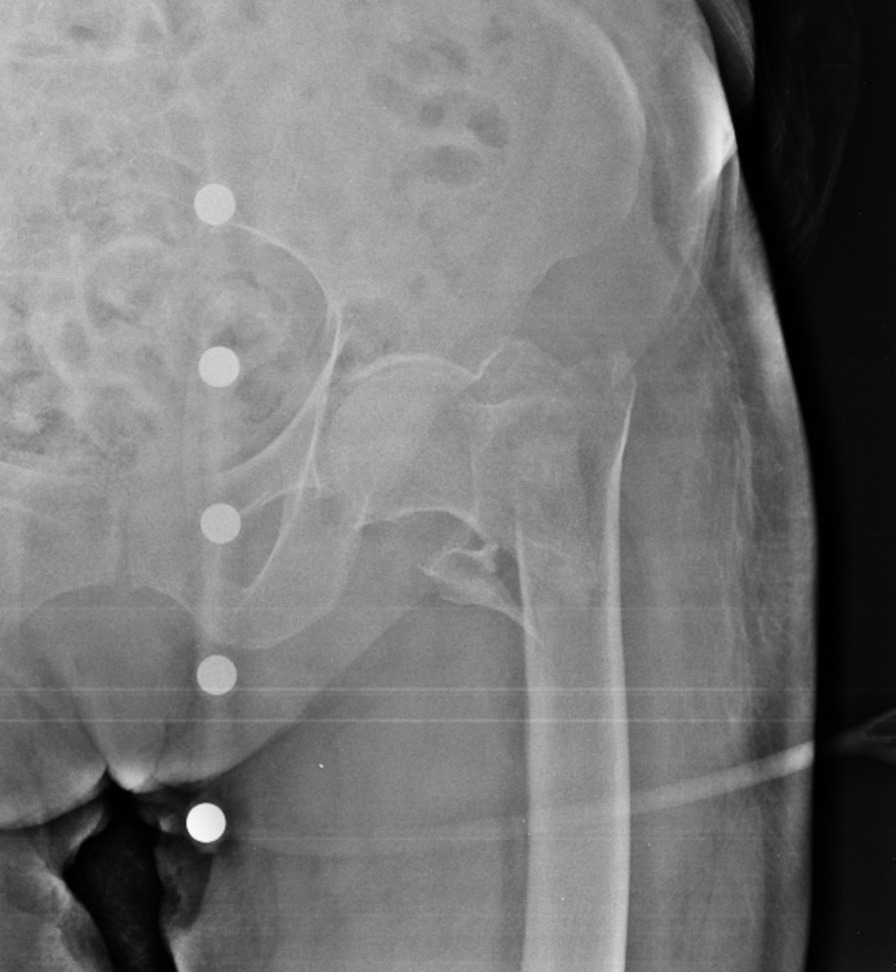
Preoperative anteroposterior radiograph of the left hip demonstrating displaced intertrochanteric femur fracture
